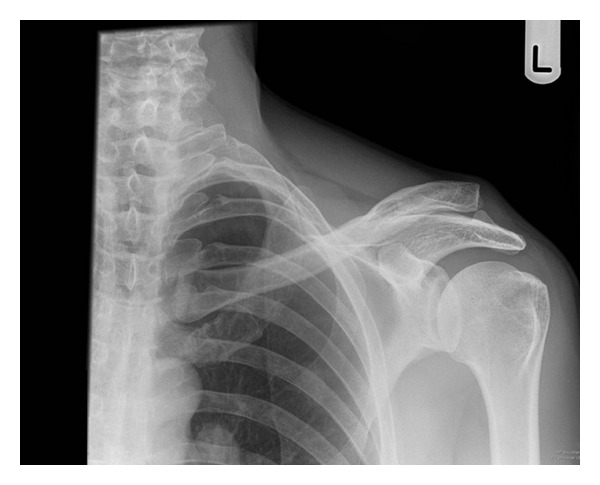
AP radiograph taken in emergency department at initial presentation.
